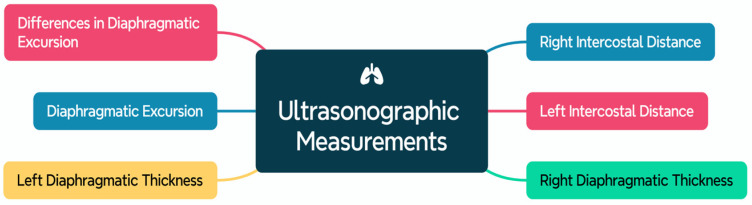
Ultrasound measurements.
